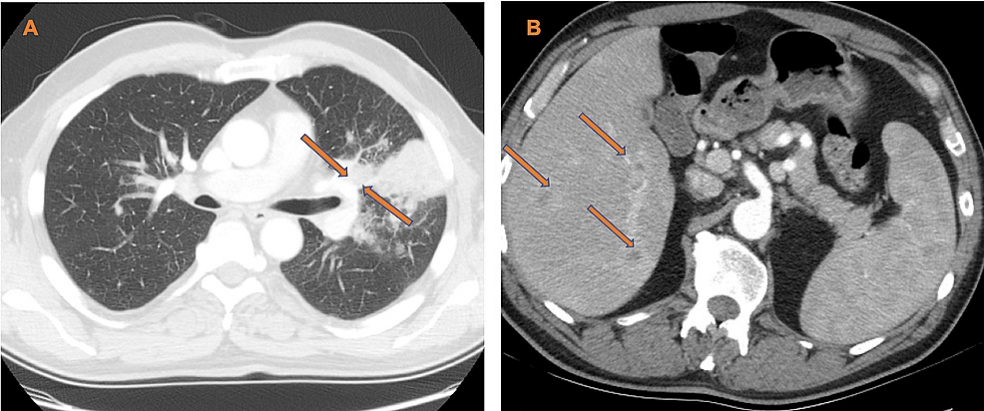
A, CT chest showing mass in left upper lobe with invasion of the left hilar structures; B, hepatic masses
